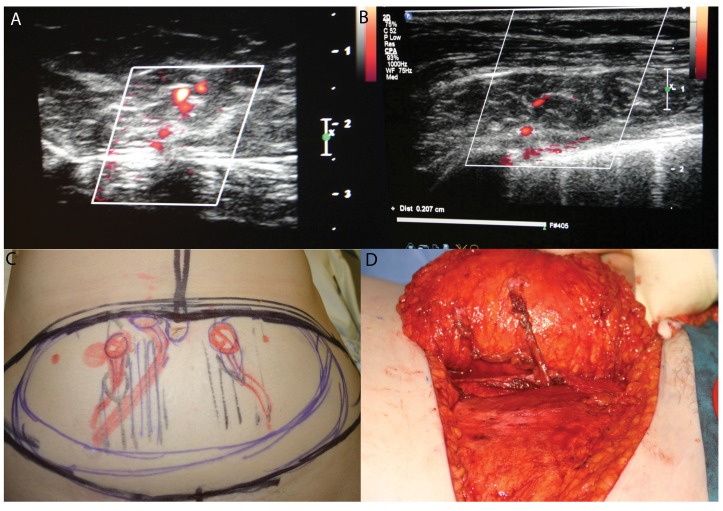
Duplex ultrasonography perforator marking: duplex images (A, B); perforator mapping on the abdomen (C); intra-operative findings (D)
